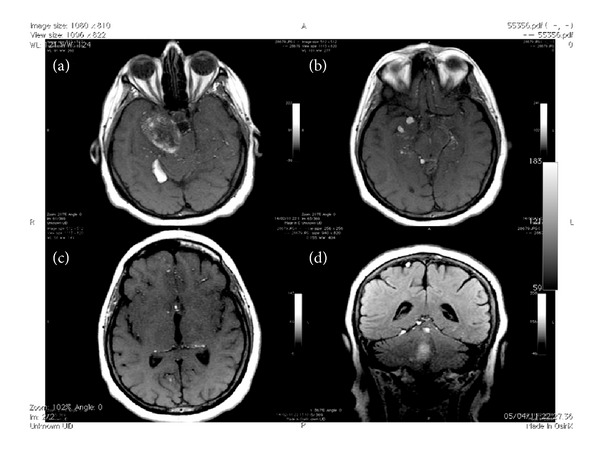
Axial SE T1-w images showed multiple bilateral micro- and macrolipid accumulations (fatty droplets ) into cerebral cisternal and cortical spaces ((a), (b), and (c)); coronal FLAIR showed hyperintense macrocollected interposed between occipital lobe and cerebellum and at the level of cerebral convexity (d).
